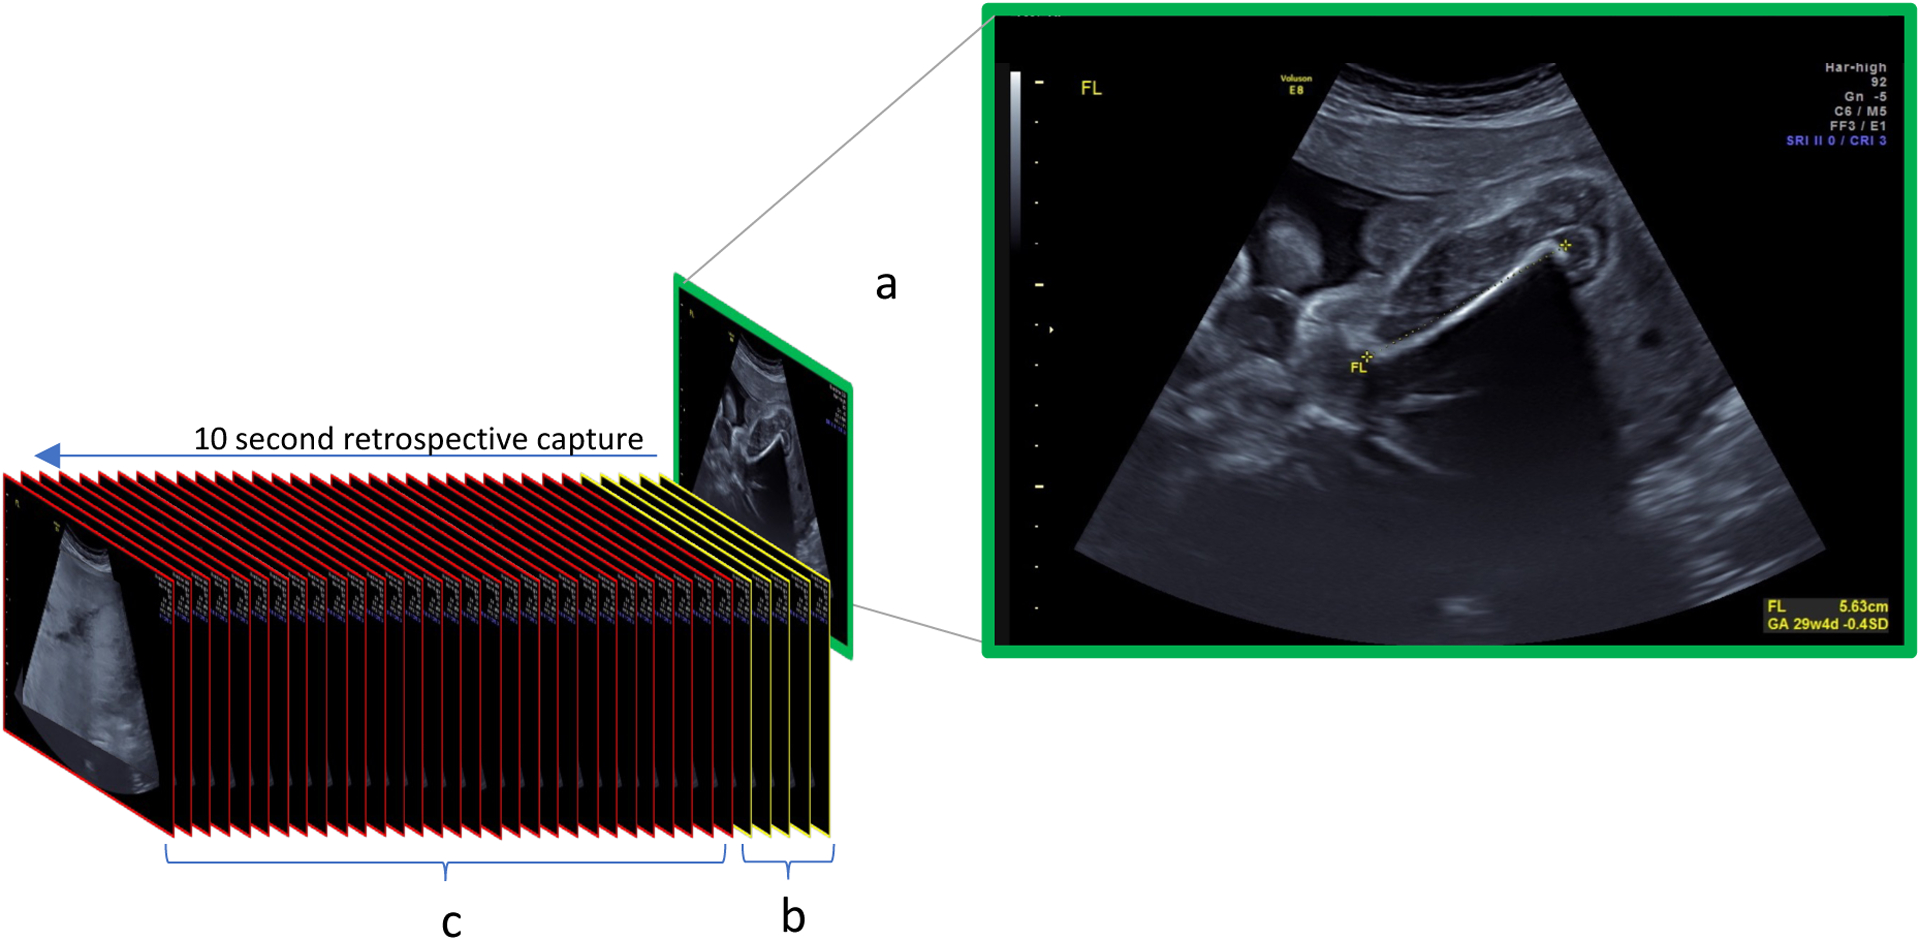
Components of a fly-to cineloop video a The last frame of a fly-to cineloop is what the sonographer freezes and measures. b The frames immediately preceding the last frame are often very similar to the last frame. c The earlier frames in a fly-to cineloop may contain other structures adjacent to the structure of interest or they may contain no usable information
